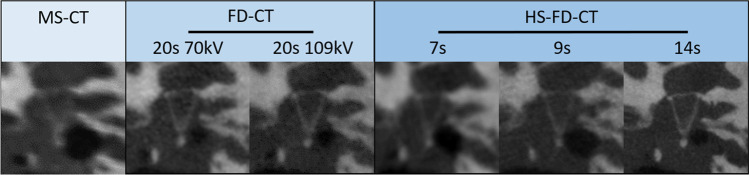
MS-CT, FD-CT, and HS-FD-CT MRPs aligned to the stapes comparing different scanning protocols
